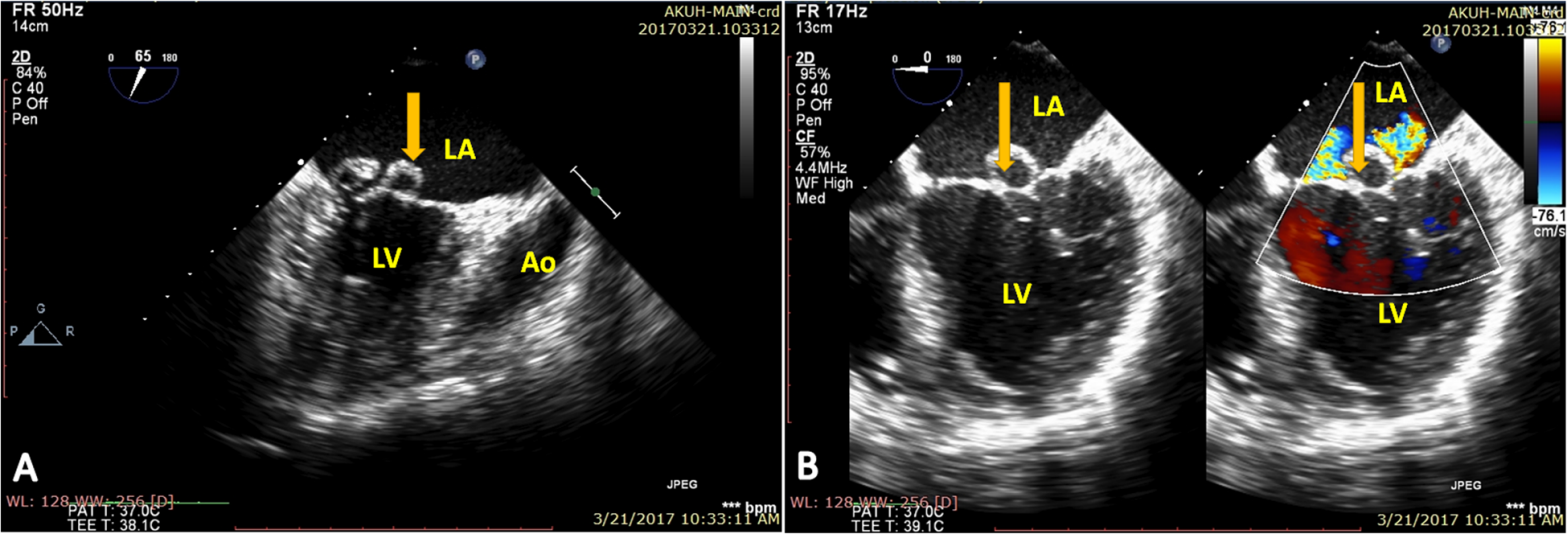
a A trans esophageal two chamber 2D echocardiogram revealing a saccular structure (yellow arrow) attached to the anterior mitral leaflet, consistent with mitral valve aneurysm. b A 2D trans esophageal echocardiogram (left) and color flow doppler (right) showing mitral valve leaflet aneurysm (yellow arrow) causing severe regurgitation. LA: Left Atrium, LV: Left Ventricle, Ao: Aorta
